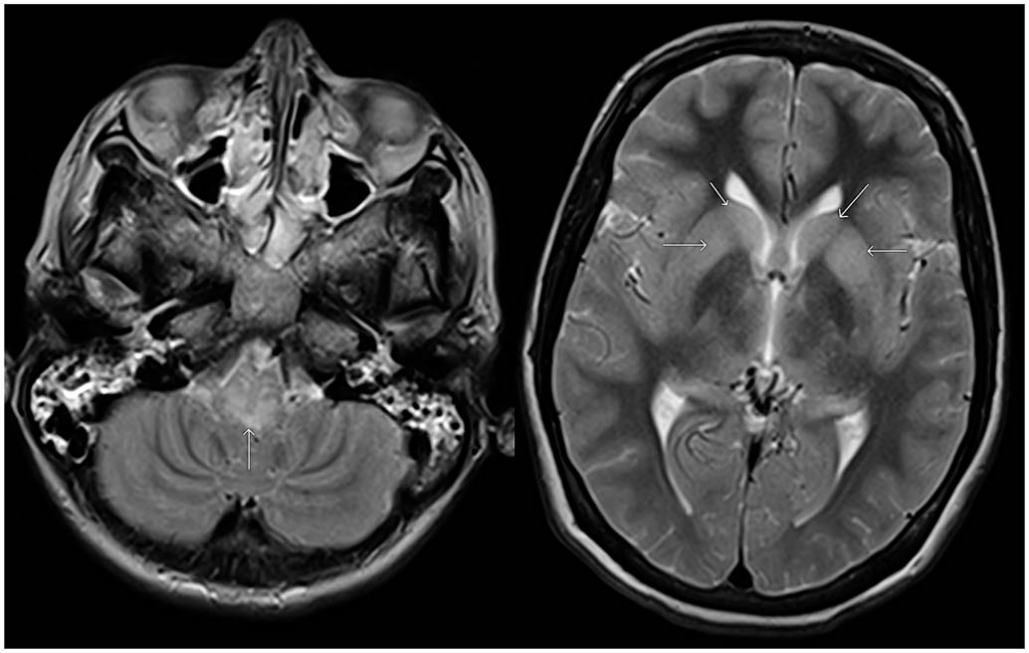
MRI brain on day 16 of admission (4 days before death). Axial T-2 weighted images in a patient with rabies due to Duvenhage virus infection, at day 17 after admission show increased signal in the posterior part of the medulla oblongata (arrow) and in the basal ganglia (arrows).
